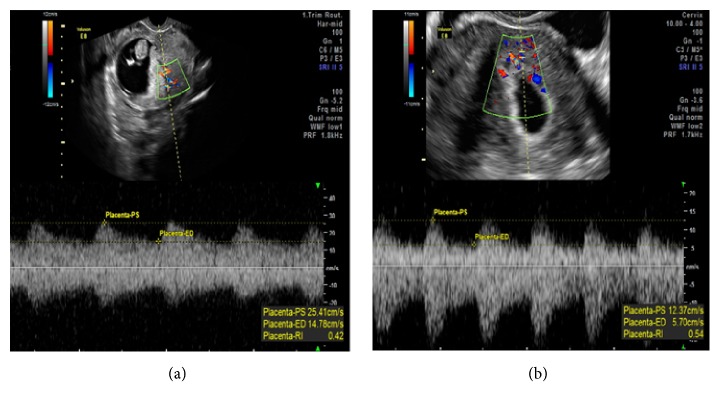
The uterine radial artery resistance index (URa-RI) was monitored with a Doppler ultrasound; (a) URa-RI women who delivered a liveborn infant and (b) URa-RI of women who miscarried the index pregnancy.
